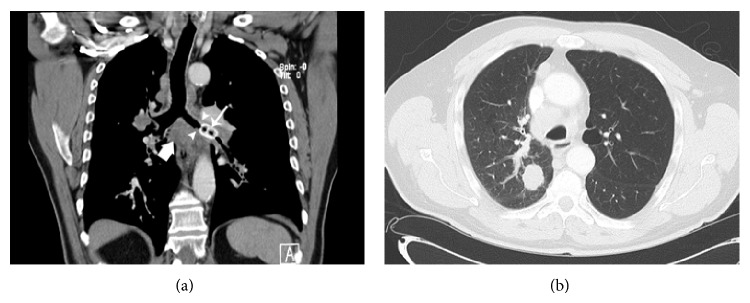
Reformatted coronal CT MIP image in soft tissue window-level setting (a) demonstrates presence of a hyperdense foreign body (solid arrow) within a thickened left mainstem bronchus (arrowheads). Also noted is subcarinal (block arrow) and hilar adenopathy which was later shown to represent nodal metastasis from lung adenocarcinoma. Axial CT imaging in lung window-level setting (b) shows resultant hyperinflation and hyperlucency of the left upper lobe related to air-trapping.
